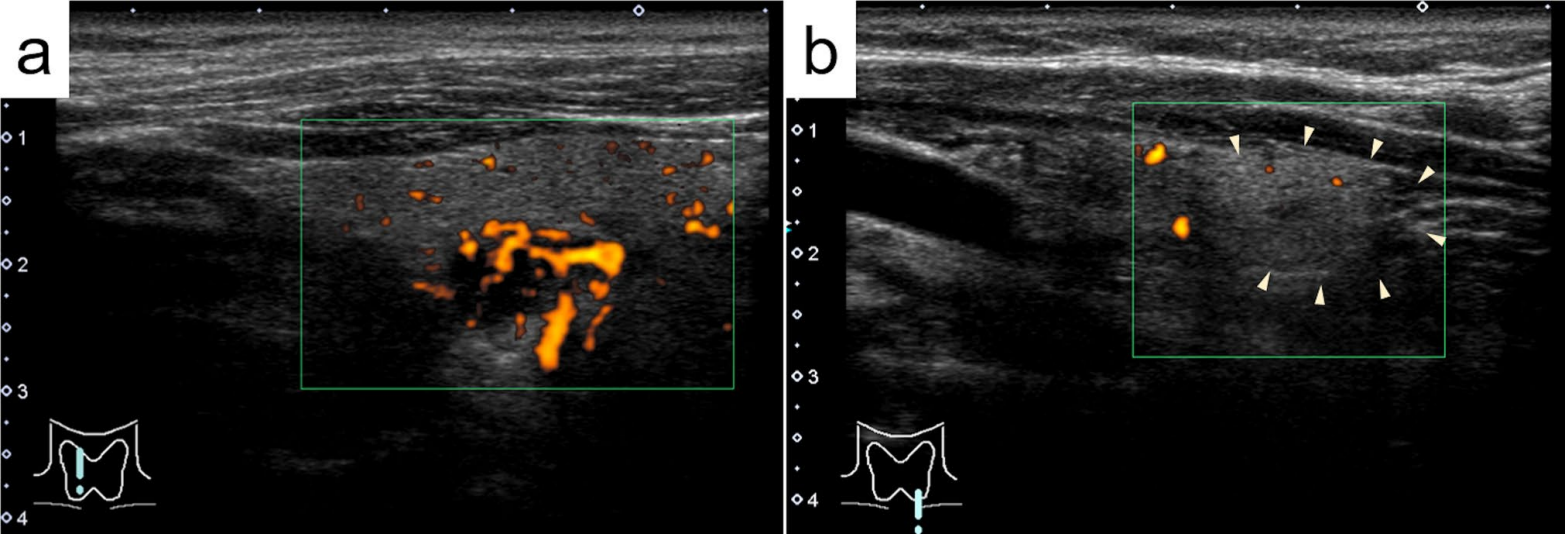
a (Group 1): A blood flow signal is present within the nodule. Note the polar artery. b (Group 4): A minimal blood flow signal is seen (power Doppler)
